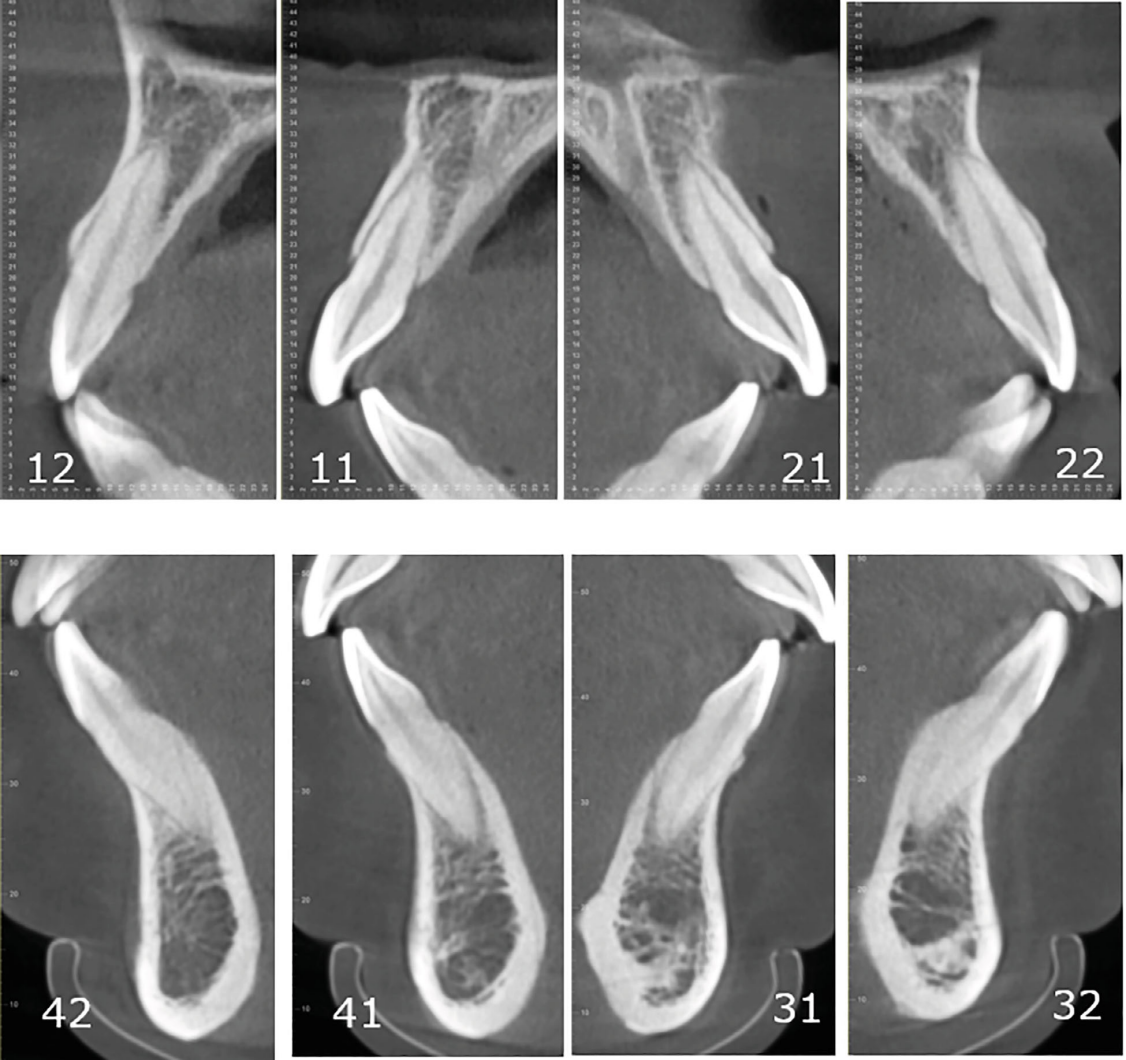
Pretreatment alveolar bone in labiolingual dimension in CBCT.
